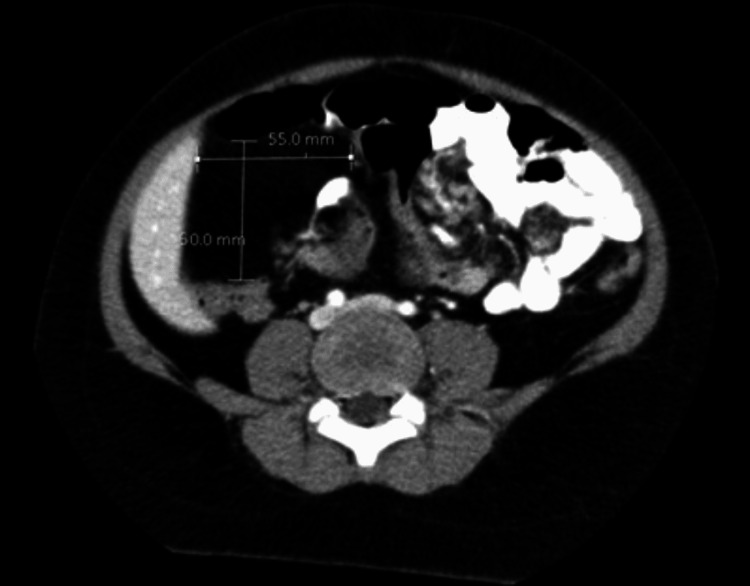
Three-month postoperative CT The image shows expanding focal area of fat in the right upper quadrant that has increased in thickness from a previous study 11 months prior, measuring 5.5 cm x 5.0 cm. There is a moderate displacement of adjacent bowel loops, raising suspicion of a growing lipoma CT: computed tomography
